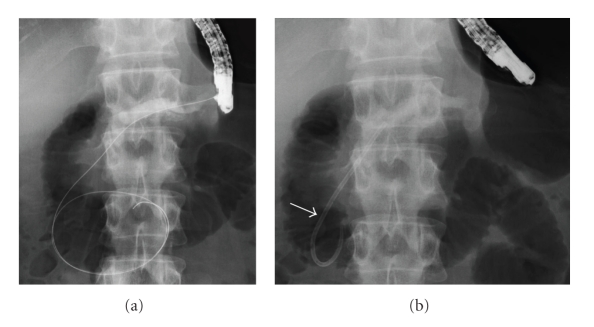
Prograde PD stenting: (a, b) X-ray image. Ten days after ESPD, a guidewire was passed through the papilla of Vater (a). A 7 F stent with a single duodenal pigtail (arrow) was inserted into the PD via the puncture tract (b).
